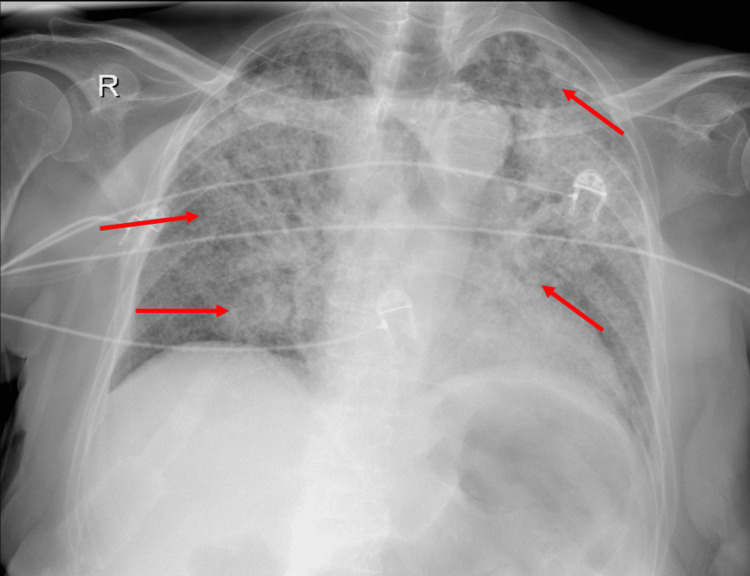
Chest radiograph: Diffuse bilateral increase in pulmonary density with patchy radio-opaque areas (red arrows) and visible air bronchograms, consistent with alveolar consolidation.
